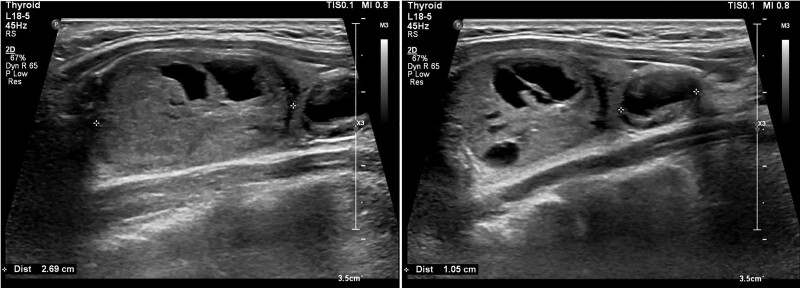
Thyroid ultrasound showing 2 nodules in the right lobe of a 13-year-old girl with autonomous functioning thyroid nodules due to somatic activating *TSHR* mutation.
